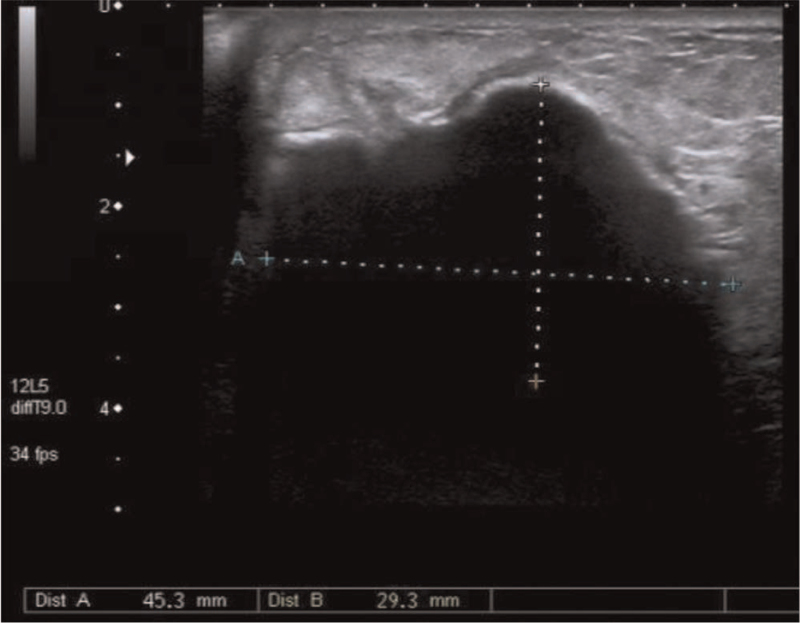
Ultrasonography. A 45.3 mm-diameter relatively smoothly marginated hypoechoic mass containing multiple calcifications.
